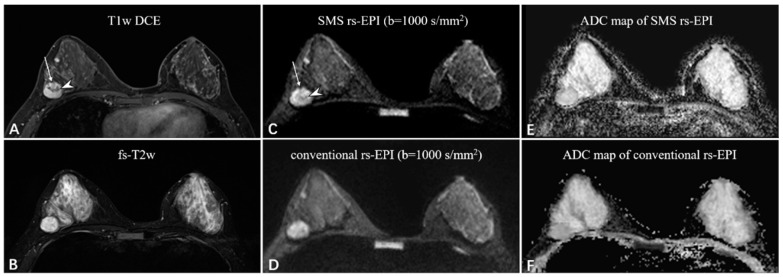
A 38-year-old woman with fibroadenoma in the right breast. The oval mass with slight lobulation (long white arrow) shows heterogeneous enhancement with dark internal septation (white arrowhead) on the T1-weighted DCE images (A) and strong high-signal intensity on the fat-saturated T2-weighted images (B). Compared to the rs-EPI image (D), the SMS rs-EPI image (C) shows better sharpness of anatomical details, in which the lobulated margin pointed by long white arrows and internal heterogeneous component pointed by white arrowheads are clearly shown. The lesion is more conspicuous with a clear margin and less distortion in the ADC map of SMS rs-EPI, although there is no ADC reduction due to the benign nature of the lesion (E,F).
